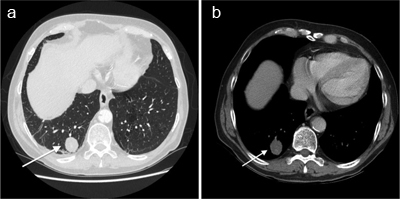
Contrast-enhanced CT scan of the abdomen shows a well-defined pulmonary nodule (27mm × 23 mm) in the right lower lobe.
